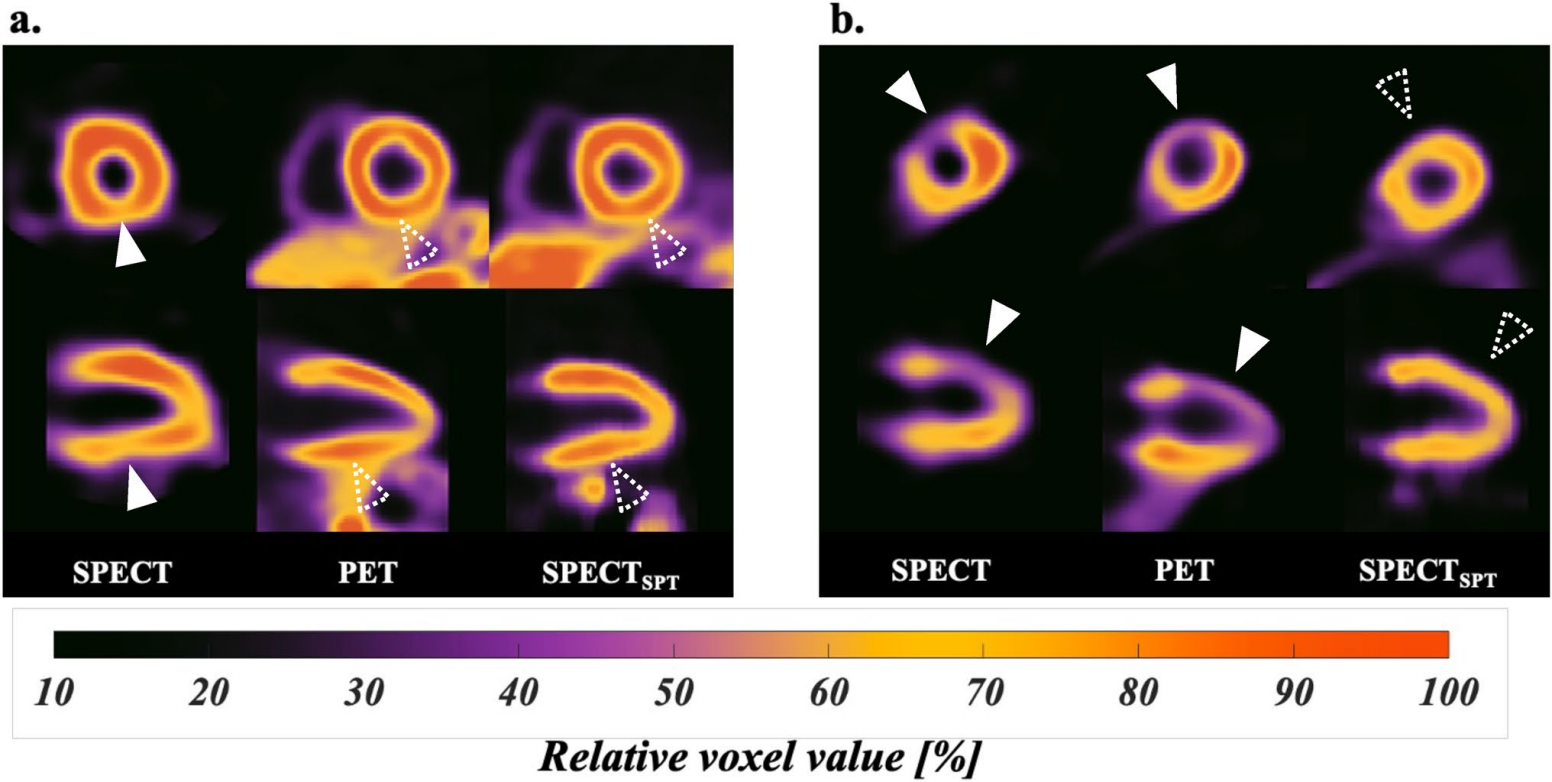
The same patient cases of SPECT, PET, and attenuation-corrected SPECT_SPT_. a Short-axis and vertical long-axis colored relative uptake images of a 54-year-old man with ventricular dilation (EDVi = 122 mL/m^2^ and ESVi = 74 mL/m^2^) but without ischemia are shown. White arrowheads indicate slightly reduced activity in the mid-inferior region on SPECT, but no reduced activity areas were found using PET and SPECT_SPT_ (white dashed line arrowheads). b Short-axis and vertical long-axis colored relative uptake images of a 50-year-old man with ischemia are shown. Significant middle to apical defect areas were observed on SPECT and PET, but the defect areas disappeared on SPECT_SPT_. EDVi, end-diastole volume index; ESVi, end-systole volume index; SPECT_SPT_, SPECT-to-PET translation model-generated SPECT; SPECT, single-photon emission computed tomography; PET, positron emission tomography
